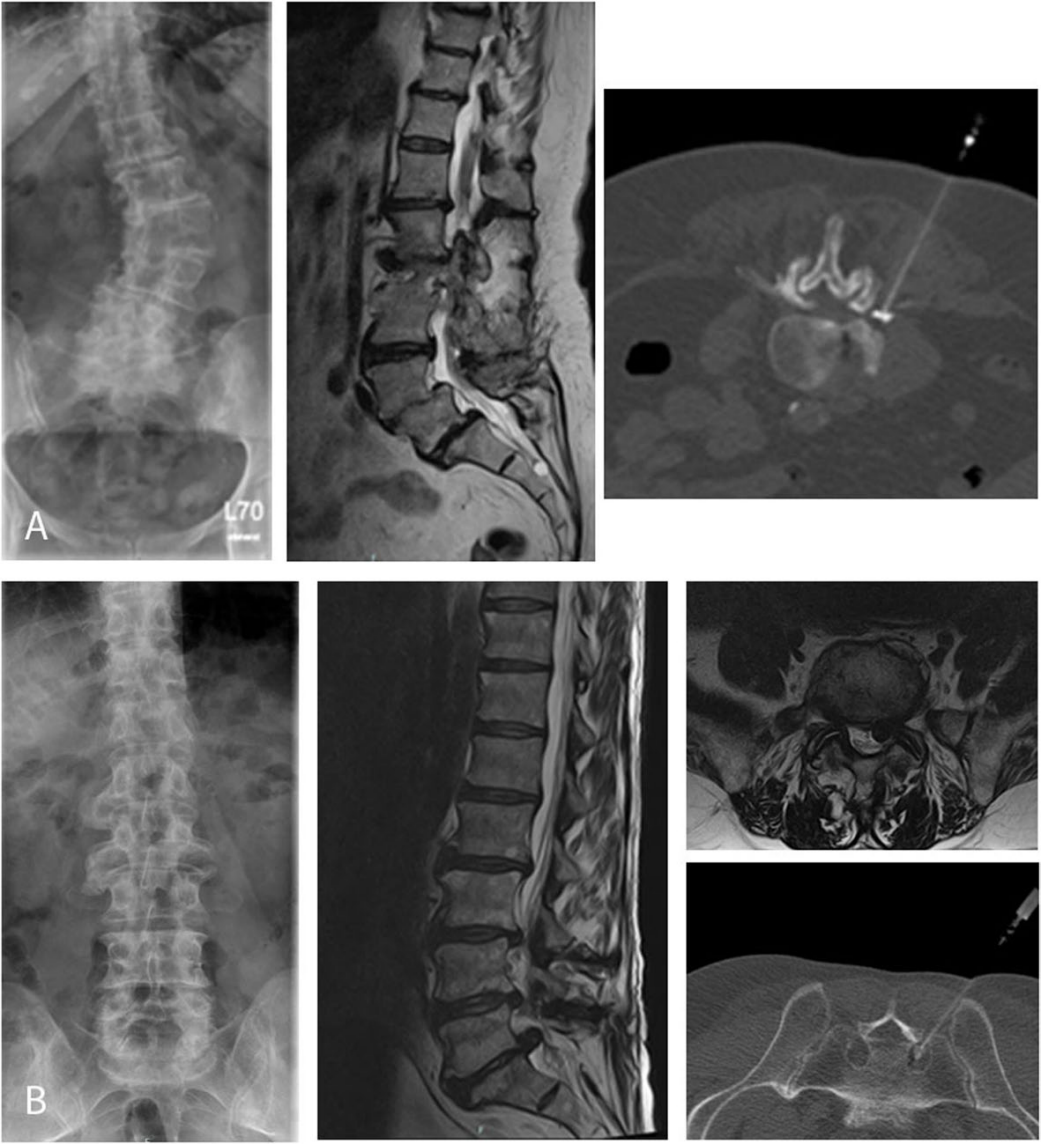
A X-ray and sagittal T2-weighted magnetic resonance imaging of degenerated lumbar spine disease with scoliosis and spondylolisthesis (75 years old woman) and ct-guided periradicular injection with contrast of the L3 nerve root on the right side. B X-ray and sagittal T2-weighted magnetic resonance imaging of lumbar spine with disc herniation L5/S1 and S1 nerve root stenosis on the left side (59 years old man) and ct-guided periradicular injection with contrast of the S1 nerve root
